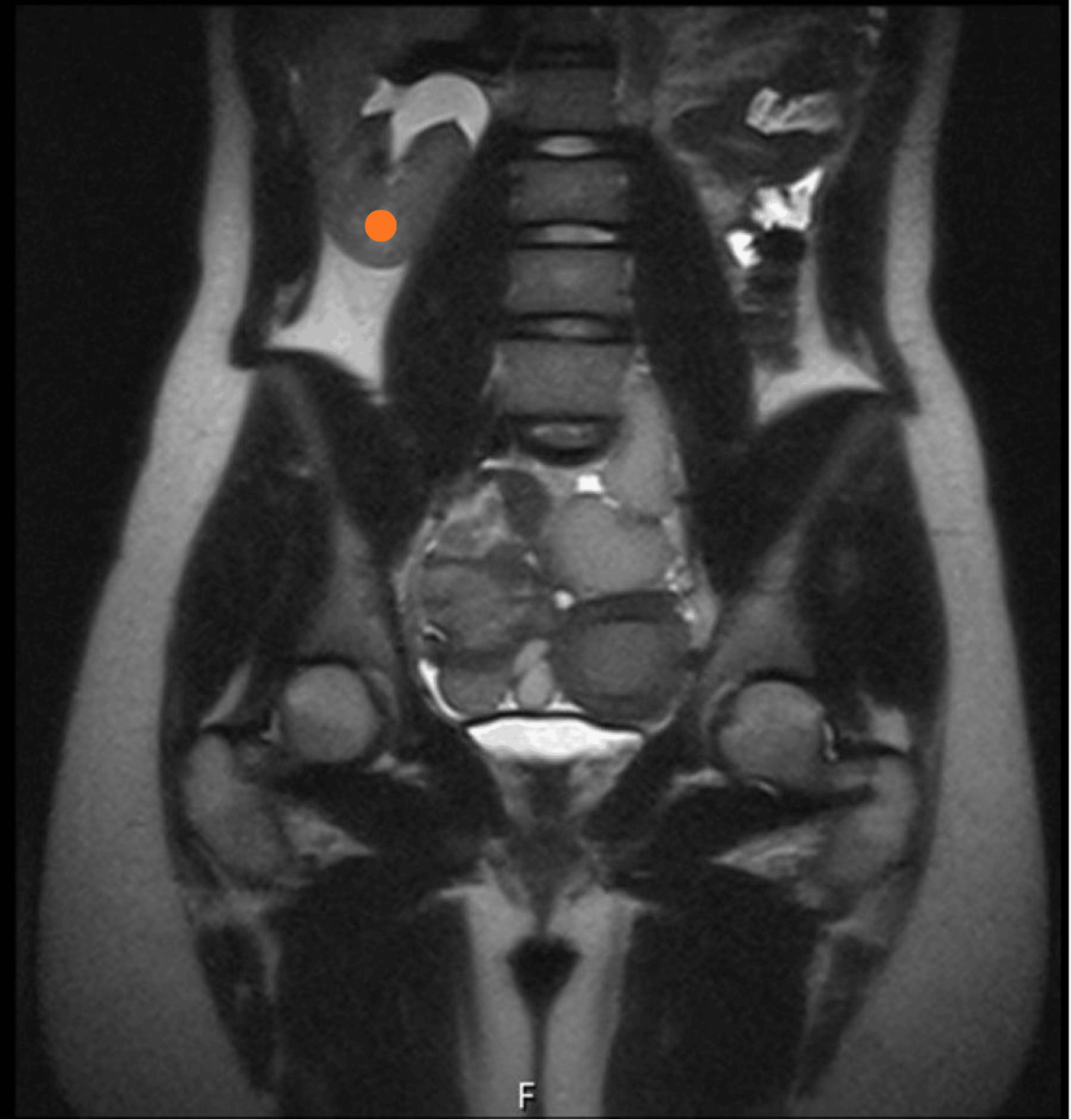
Coronal T2 MRI Orange dot: right compensatory kidney MRI: magnetic resonance imaging
